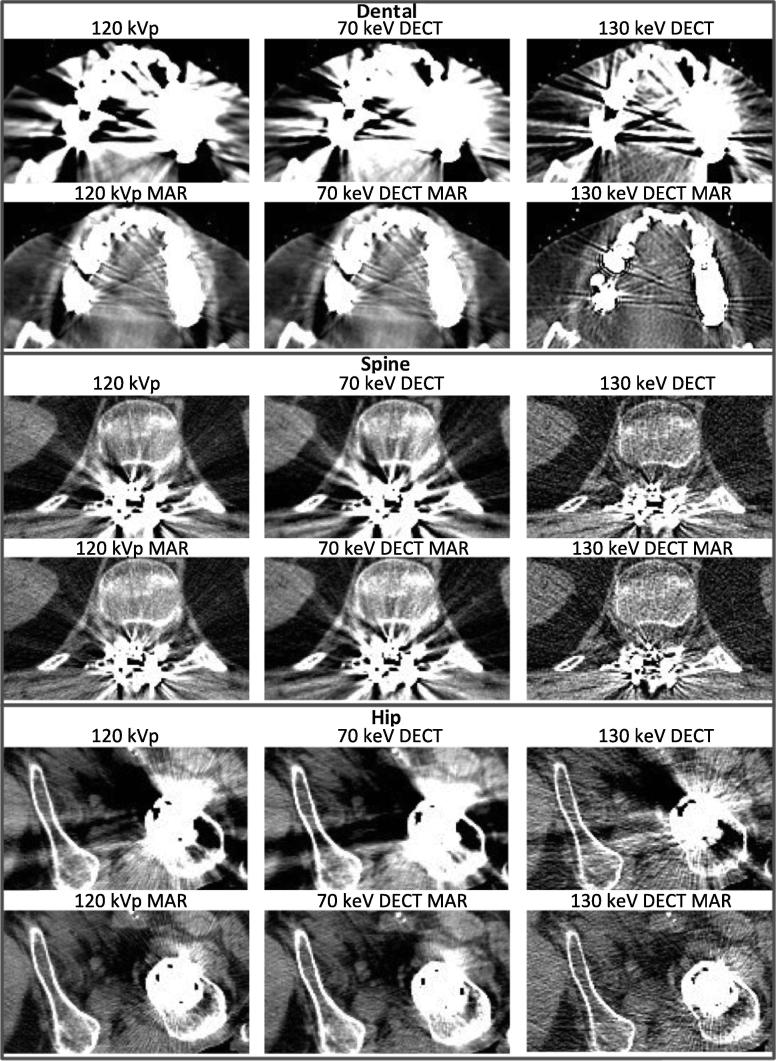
Three patients shown in standard acquisition scan for radiotherapy treatment planning (120 kVp) and in the five studied reconstruction modalities (70 keV DECT, 130 keV DECT, 120 kVp MAR, 70 keV DECT MAR and 130 keV DECT MAR). Two radiologists and three oncologists performed pairwise comparisons to rank the images. For contouring in the artefact affected area, observers preferred the combination of dual energy CT virtual monochromatic extrapolation at the 130 keV level and the iterative metal artefact reduction algorithm (130 keV DECT MAR) in the presence of artefacts from dental and spine implants, while 120 kVp MAR was preferred in the presence of artefacts from hip implants.
